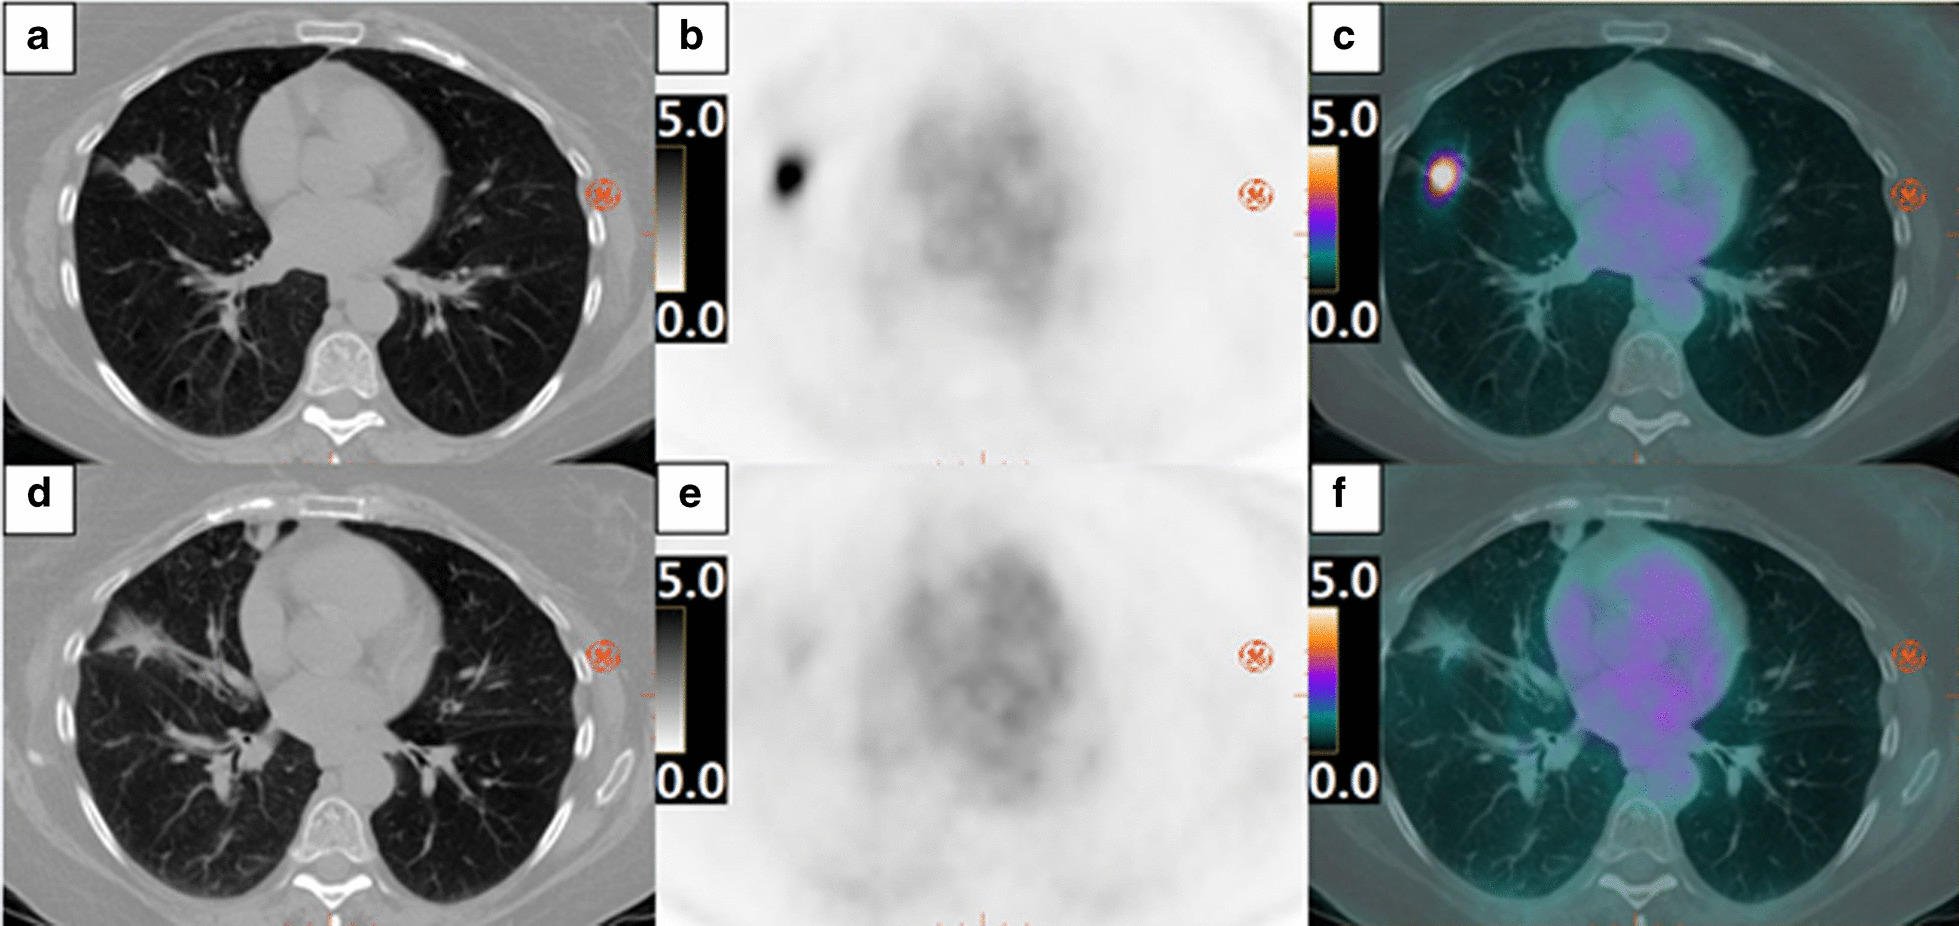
Example of RECIST Failure. Pre-SABR images of pCR patients on CT, PET SUV (range 0–5.0 g/mL), and PET/CT fused are shown in a, b and c, respectively. Post-SABR CT, PET and PET/CT fused images are shown in d, e and f, respectively. *RECIST* Response Evaluation Criteria in Solid Tumours 1.1, *SABR* stereotactic ablative radiation therapy, *pCR* pathologic complete response, *CT* computed tomography, *PET* positron emission tomography, *SUV* standardized uptake value
